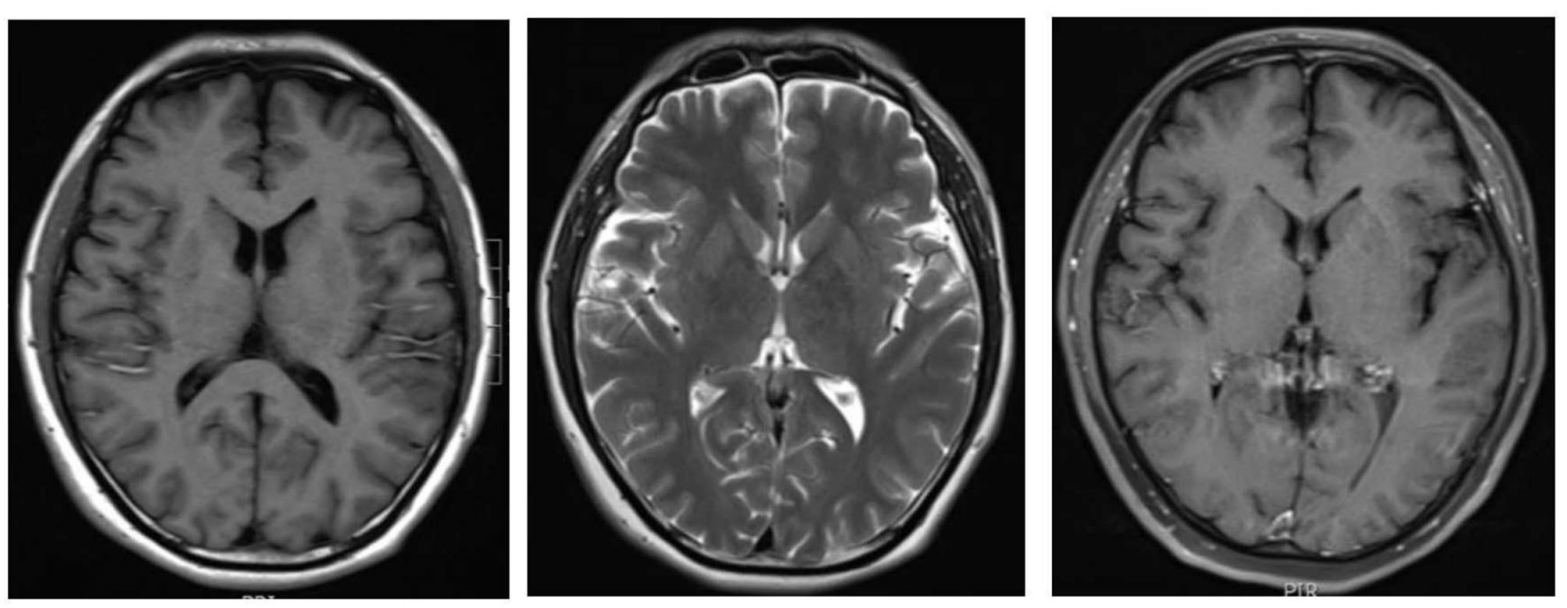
Brain magnetic resonance imaging done on the first hospital day. T1WI/T2WI/Post-Contrast T1WI didn't show any abnormality.
